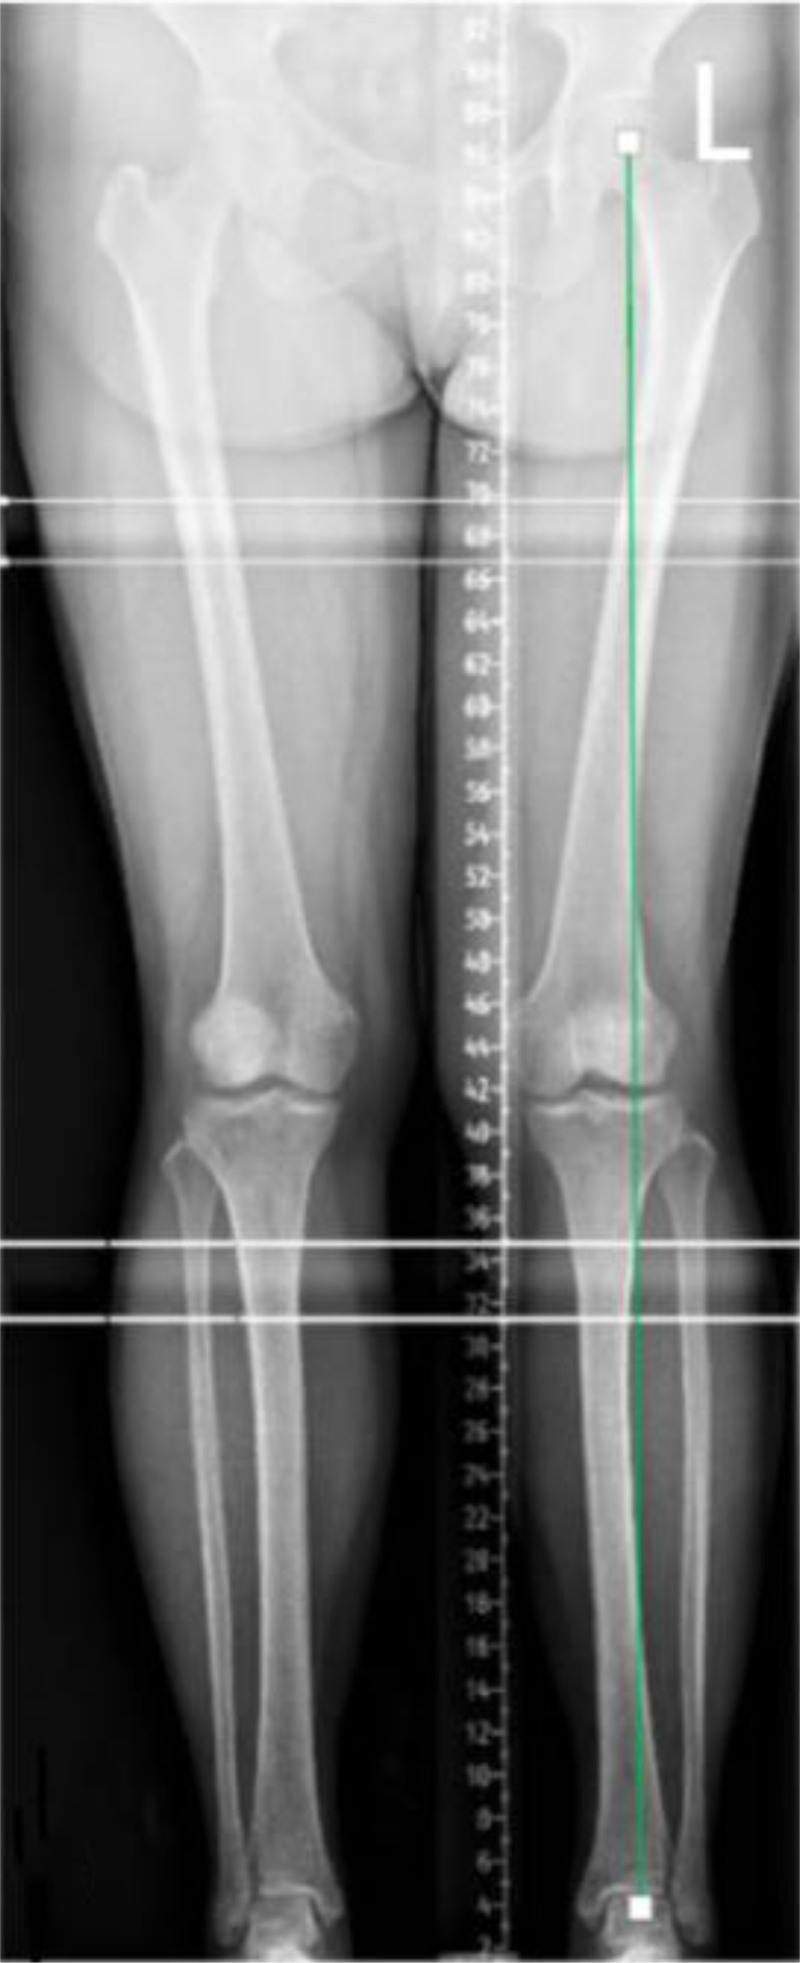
(A–D) Postoperative (4-mo) MRI of the left knee. (A and B) Sagittal and coronal views showing intact ACL graft with appropriate tension. (C and D) Axial and coronal views showing intact MPFL graft with appropriate tension. MPFL = medial patellofemoral ligament, MRI = magnetic resonance imaging.
